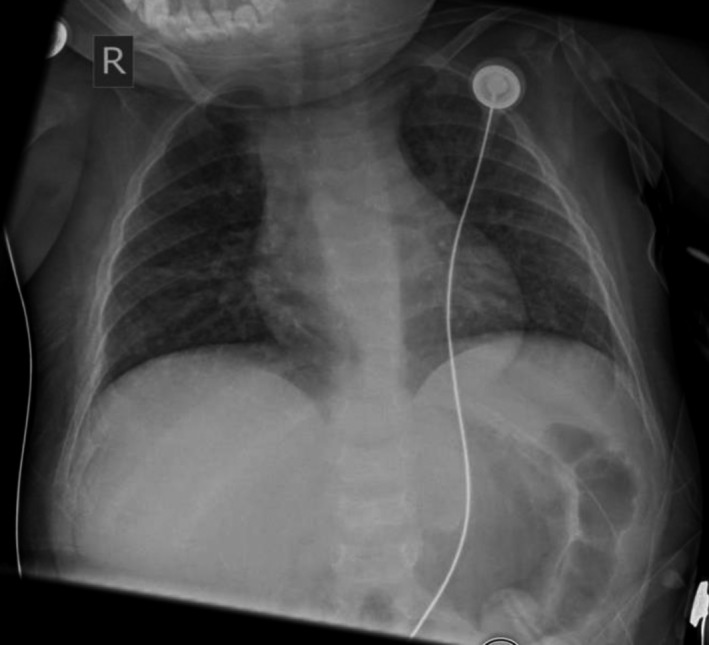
Chest radiograph 5 months prior to this hospitalization with chronic interstitial pulmonary changes.
